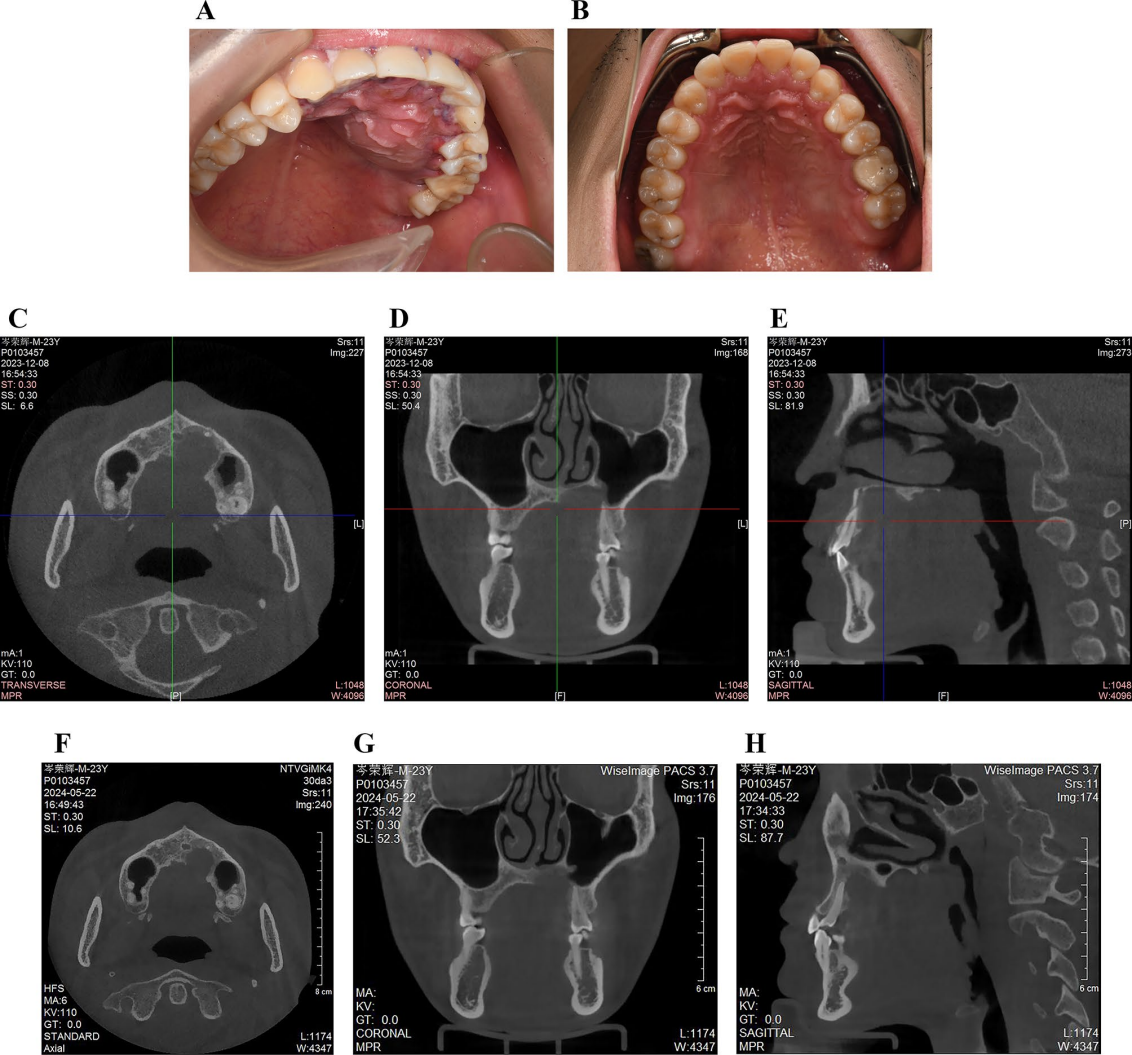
Postoperative data. A. An intraoral photograph presenting the swelling in operative area after one week postoperatively. B. Normal findings in the clinical presentation after 3 months. C, D, and E. The CBCT images showed some amount of bone formation with no sign of recurrence after 3-month follow-up. F, G, and H. The 9-month follow-up CBCT images indicated no evidence of recurrence
